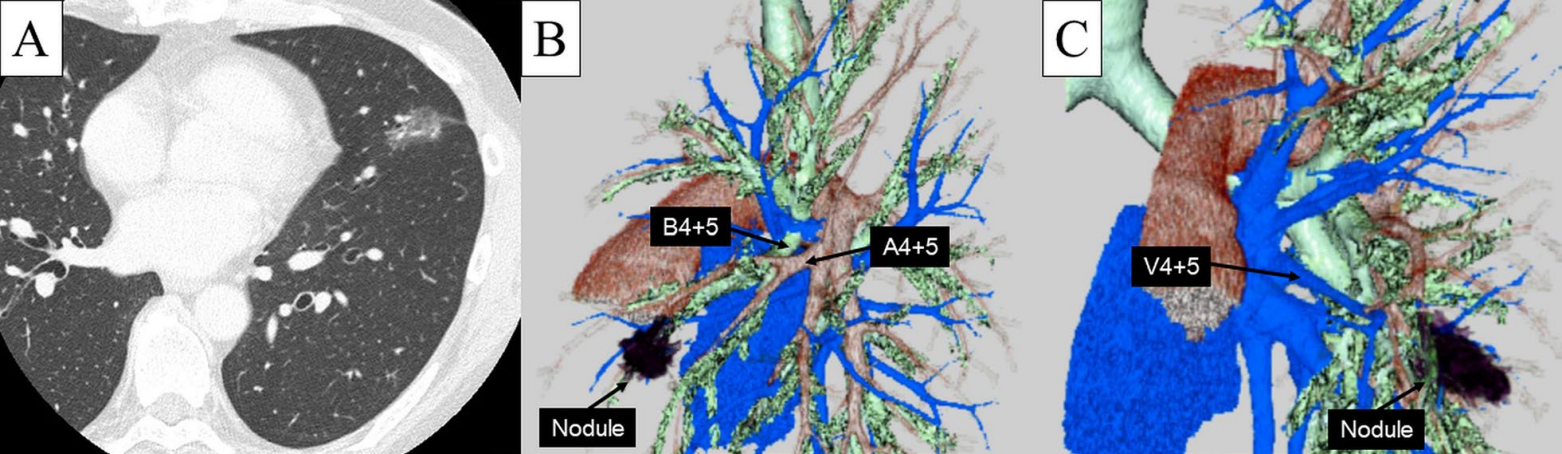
Preoperative chest computed tomography image and three-dimensional images. A Chest computed tomography showed a part-solid ground-glass nodule containing a solid component that was highly suspicious for malignancy. B The lingular pulmonary artery (A4 + 5) completely branched from the interlobar pulmonary artery. C The lingular pulmonary vein (V4 + 5) drained into the common trunk of the left pulmonary vein
